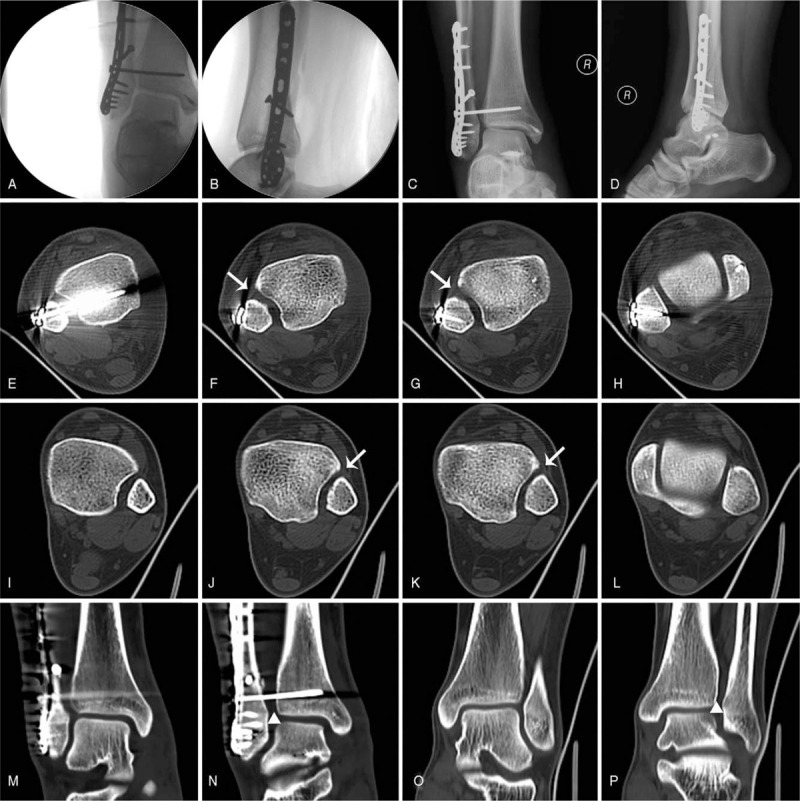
(A,B) The intraoperative fluoroscopy view. (C,D) Anteroposterior and lateral X-ray image 1-week post-operation. The distal tibiofibular syndesmosis was wider than intraoperation, notice the syndesmotic screw had a tendency to loosen. (E–L) The cross sections of CT views of bilateral side. The syndesmosis (white arrow) and mortise space (H,L) of injured side were slight wider than contralateral side. (M–P) The coronal sections of CT views of bilateral side. The syndesmosis (white triangle) and mortise space (M,O) of injured side were slight wider than contralateral side.
